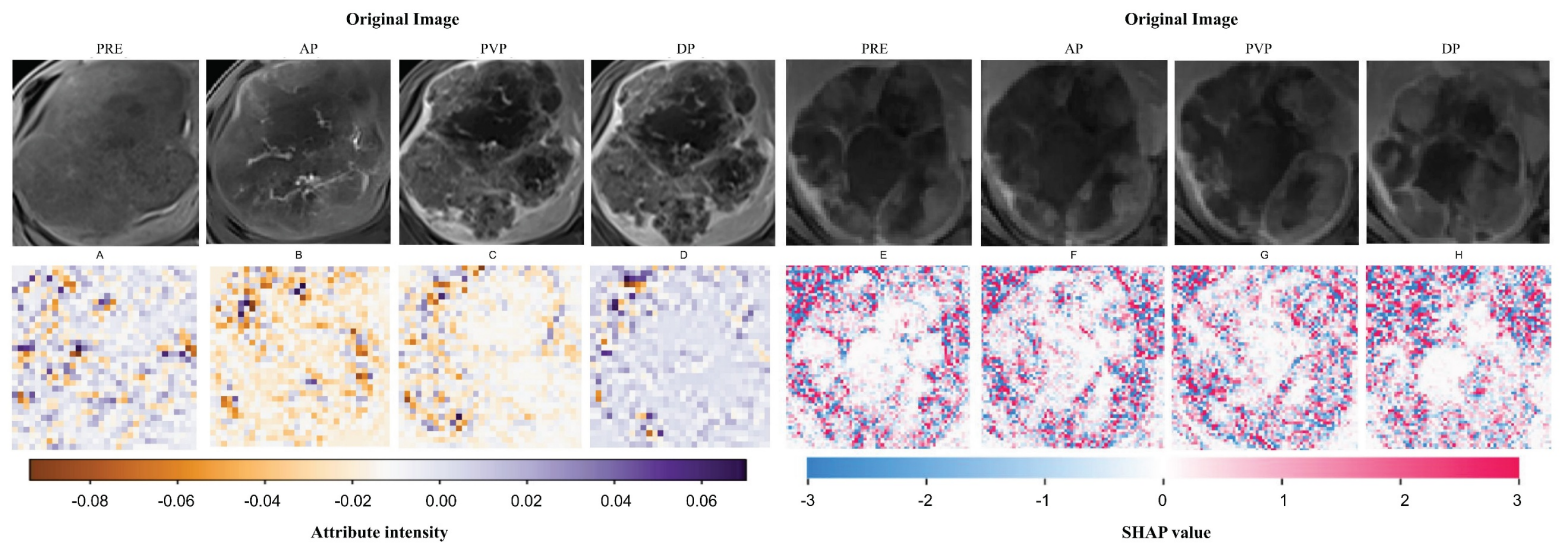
Visualization of feature mappings derived through ResNet50. A-D Visualization analysis of Patient A's full-sequence CE-MRI was conducted using the Grad-CAM algorithm. E-H Visualization analysis of Patient B's full-sequence CE-MRI was conducted using the SHAP algorithm. The full sequence is ordered as PRE, AP, PVP and DP.
